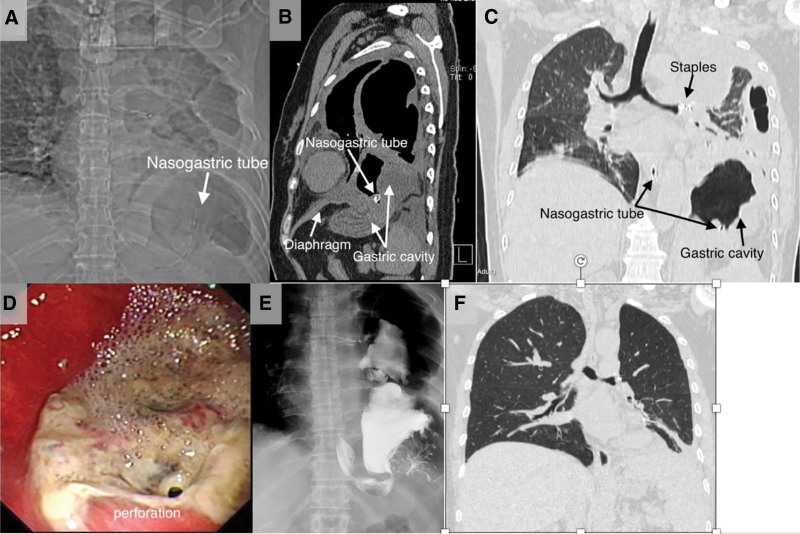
(A) The nasogastric tube was in a reverse “J” shape. (B/C) Gastric cavity passing through diaphragmatic defect. Left lung injury with pneumothorax. Partial stomach hernia into the chest cavity. (D) Gastric fundus perforation. (E) Upper gastrointestinal iohexol radiography revealed dissemination of the contrast medium in the thoracic cavity. (F) CT scan 3 months postoperatively revealed well recovery. CT = computerized tomography.
